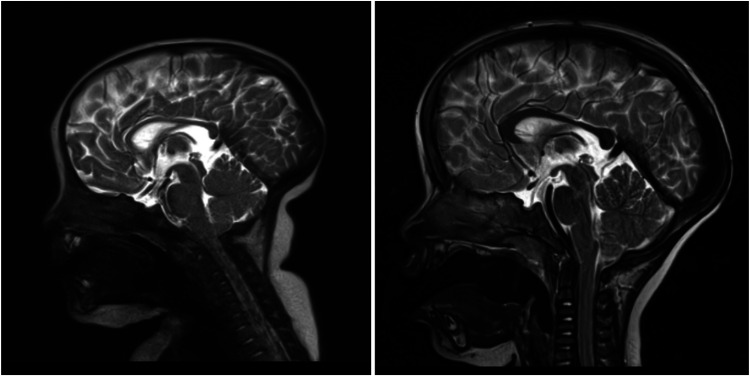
Preop (Left) and Post-Spring Removal (Right) MRI.
